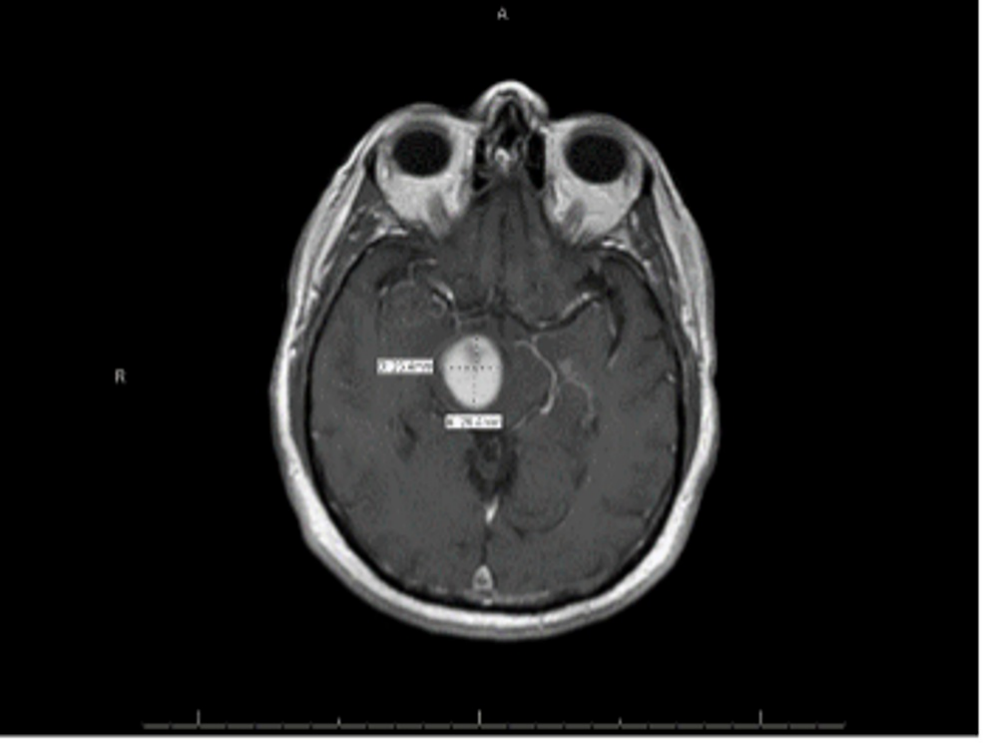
MRI findings at the time of presentation – homogenously enhancing solitary mass centered in the right midbrain and right cerebral peduncle measuring 2.3 cm × 2.6 cm × 2.5 cm
